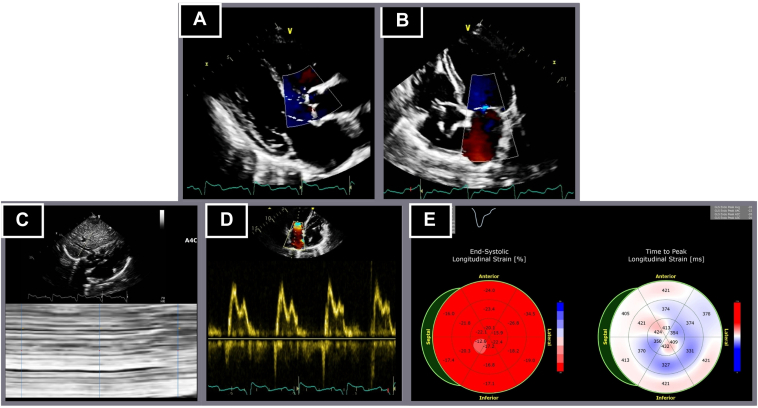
Control transthoracic echocardiogram. Left ventricular ejection fraction of 55%. A: Parasternal long axis view. B: Apical 4-chamber view. C: Collapsed inferior vena cava. D: Transmitral flow Doppler shows peak E and peak A waves previously absent. E: Normalized longitudinal strain of −20.1%.
